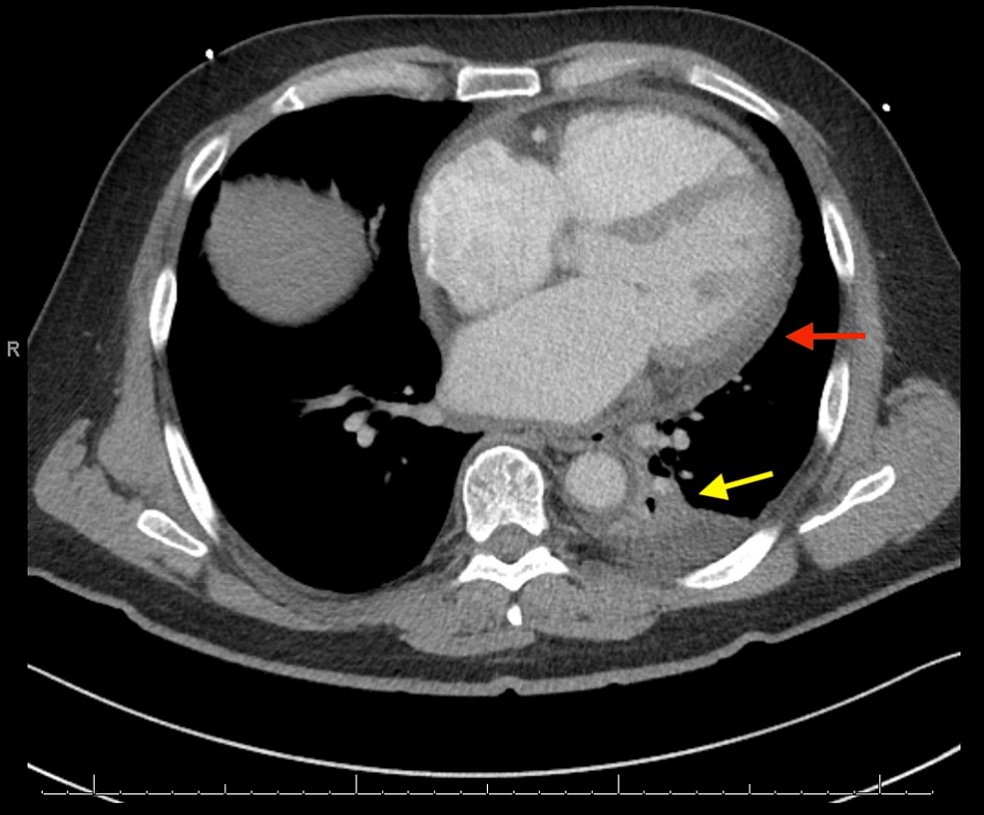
CT chest showing a small pericardial effusion as well as a left lower lobe infiltrate Red arrow refers to pericardial effusion; yellow arrow refers to left lower lobe infiltrate.
